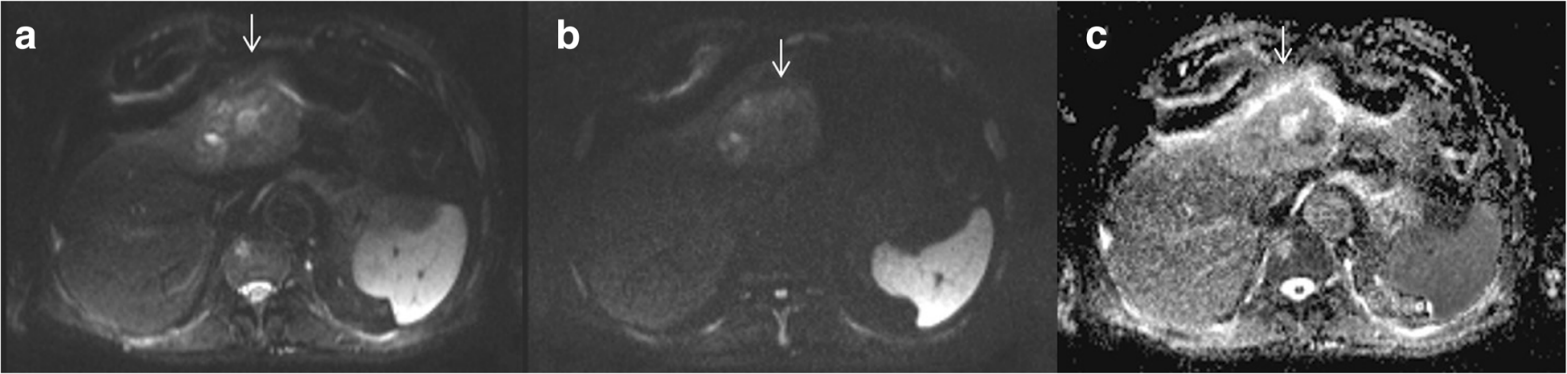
High-grade HCC. DWI sequences: in A b50 s/mm^2^; in B b800 s/mm2 and in C ADC map
